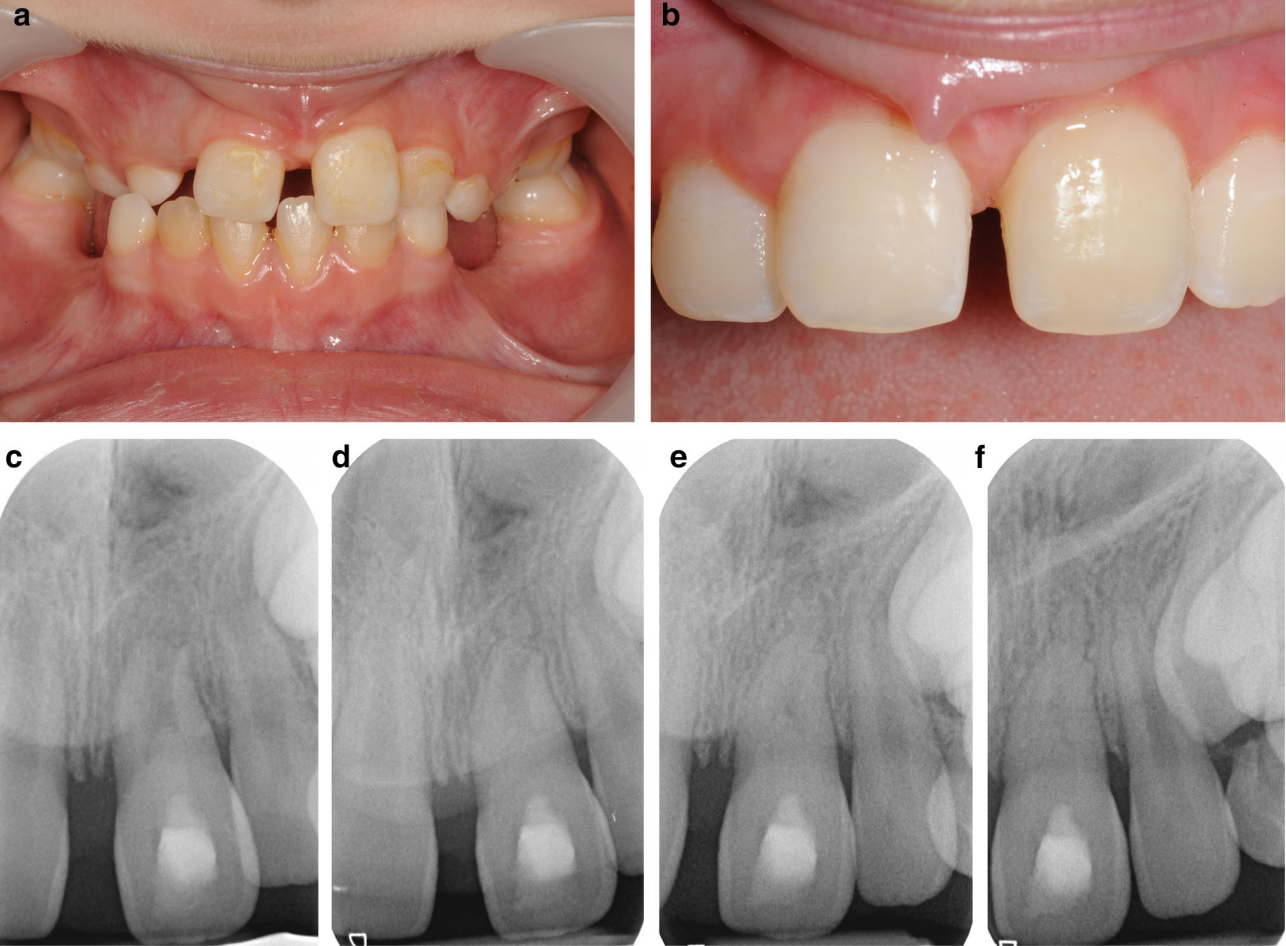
Clinical photographs (a before treatment, b 2 years following RET) and radiographs (c baseline immediately after treatment, d 3 months, e 9 months, f 2 years following RET) of an avulsed and replanted immature 21 treated with RET. No signs of infection were evident after 2 years with continuation of root developmemt, minimal replacement resorption and no signs of infra-occlusion seen at 2 years follow-up
